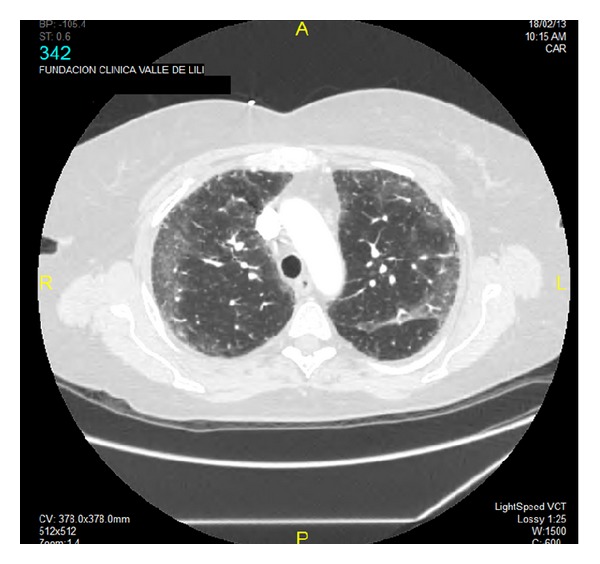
Chest computed tomography showing diffuse reticular interstitial infiltrates with ground-glass pattern of nonspecific interstitial pneumonia in a rheumatoid arthritis patient with* Pneumocystis jirovecii *pneumonia.
